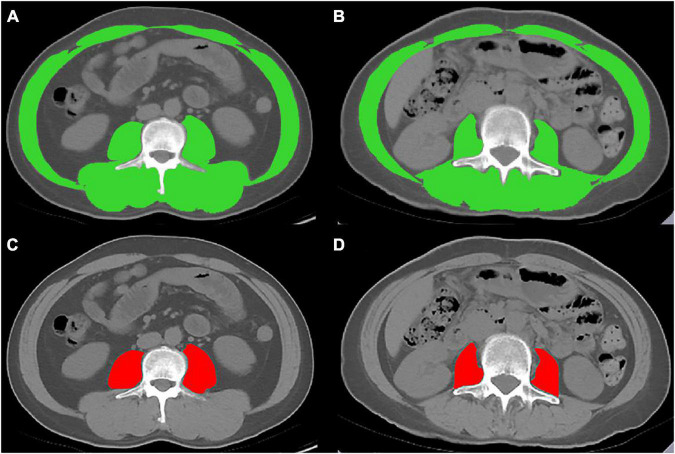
Cross-sectional computed tomographic images at the third lumbar vertebrae level. The skeletal muscle area (SMA) was measured by manual tracing in (A) male and (B) female. The psoas muscle area (PMA) was measured by manual tracing in (C) male and (D) female.
